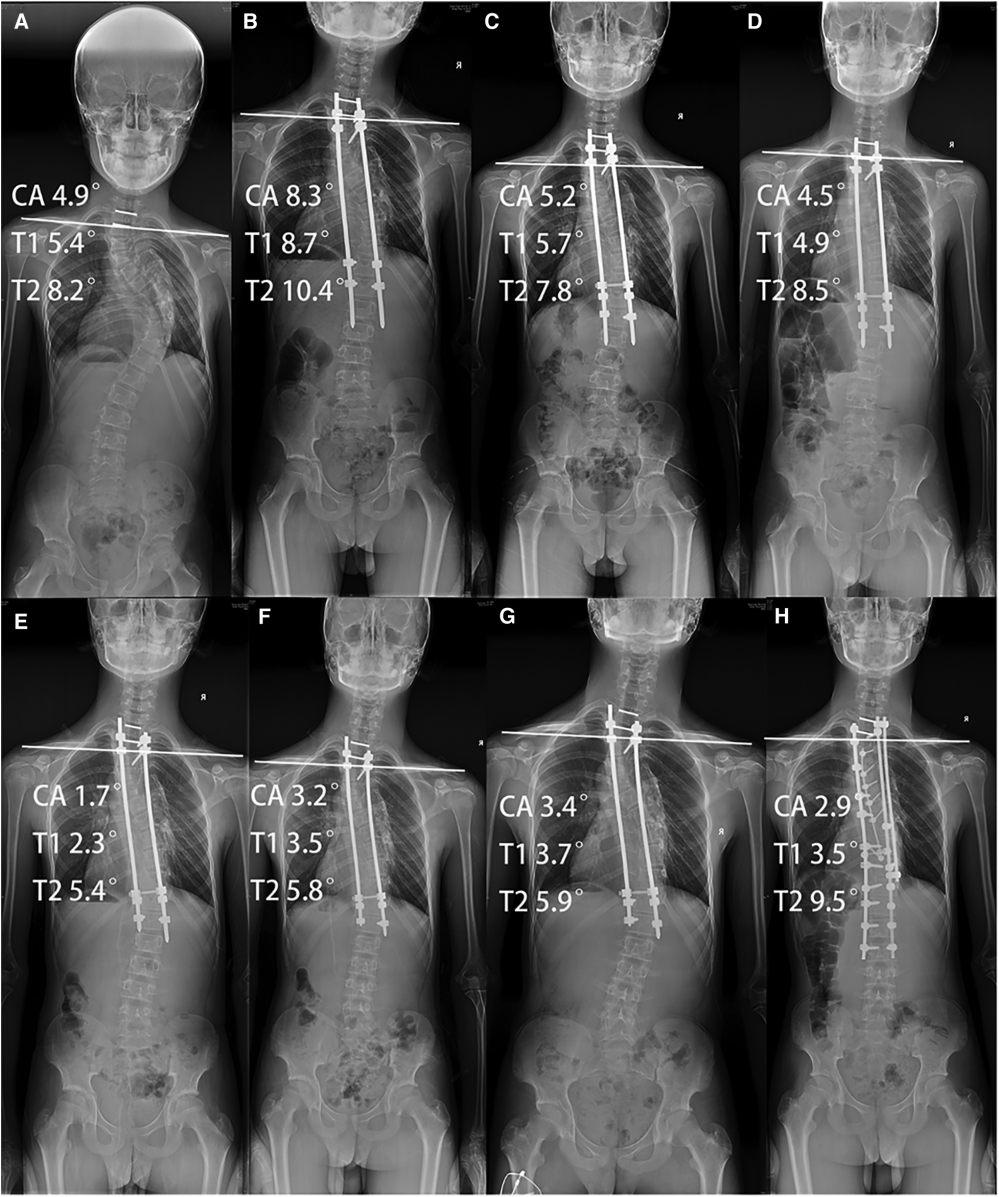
(A) Represent the preoperative entire spine radiograph of patients with the EOS patient, (B–G) represent entire spine radiograph after each growth rod extension surgery, and (H) represent the entire spine radiograph after the last growth rod extension and spinal fusion fixation surgery.
